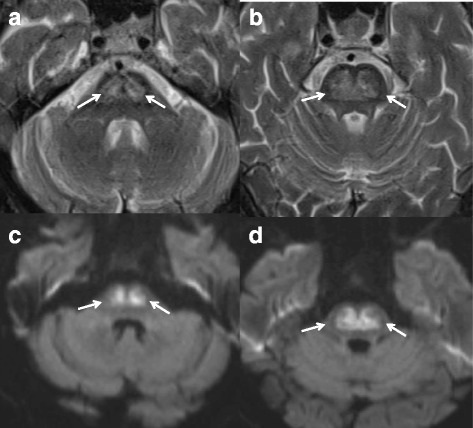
MRI images of the brain (a and b: T2-weighted, c and d: B = 1000 DWI); arrows indicate the central pontine-demyelinization area
